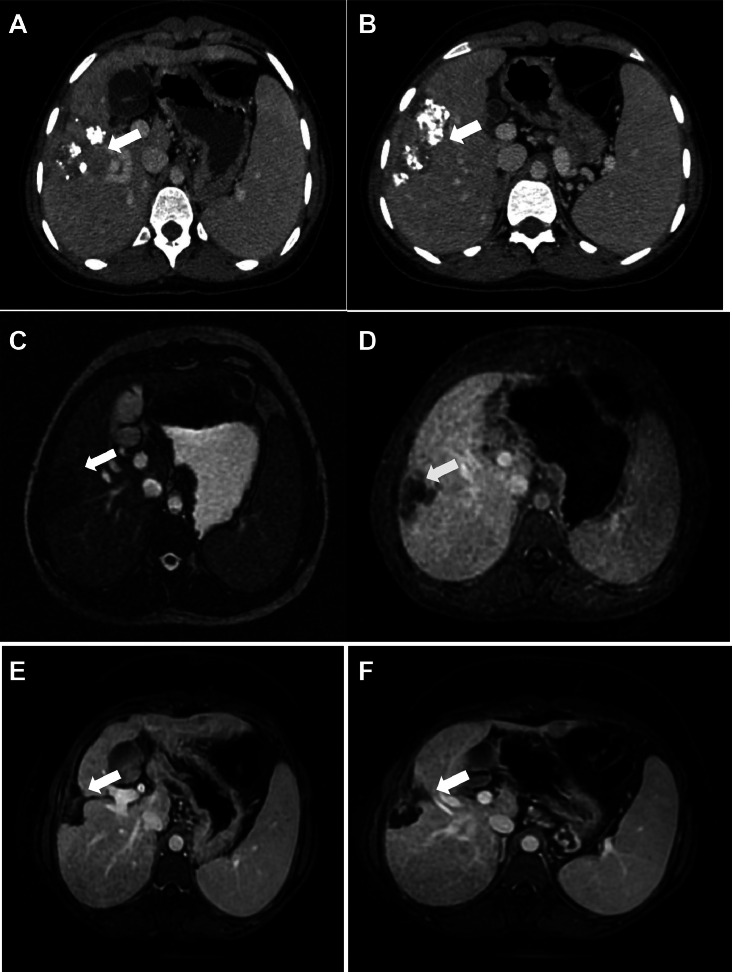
Long-term follow-up (2017-2024): Axial CT scans (A, B), MRI sequences; 2D FIESTA (C) and T1 GADO (D, E, F) showing a persistent lipiodol deposition in treated areas; no evidence of viable tumor; signs of capsular retraction; no recurrence after second embolization (white arrow).
